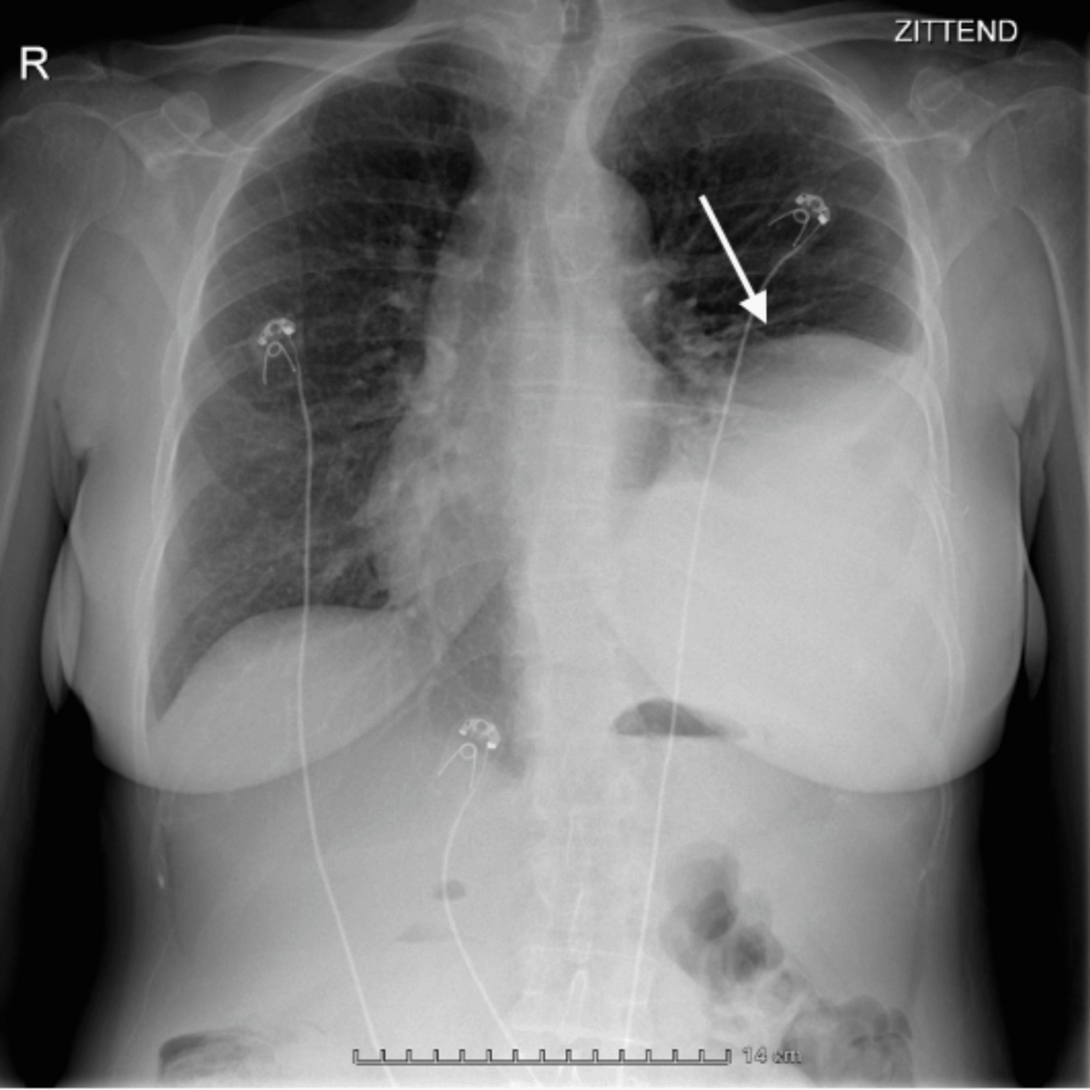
Chest X-ray on admission showed elevation of the left hemidiaphragm.
